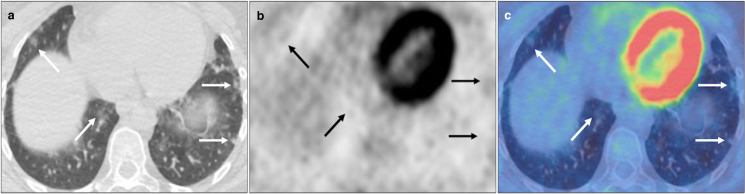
Small bilateral subpleural nodular foci of GGO demonstrating low-grade FDG uptake (solid black and white arrows), *e.g*. left lower lobe (SUV_max_ 1.4) on axial CT, PET and fused PET-CT (A-C). BSTI 3 with positive RT-PCR (*confirmed* case).
